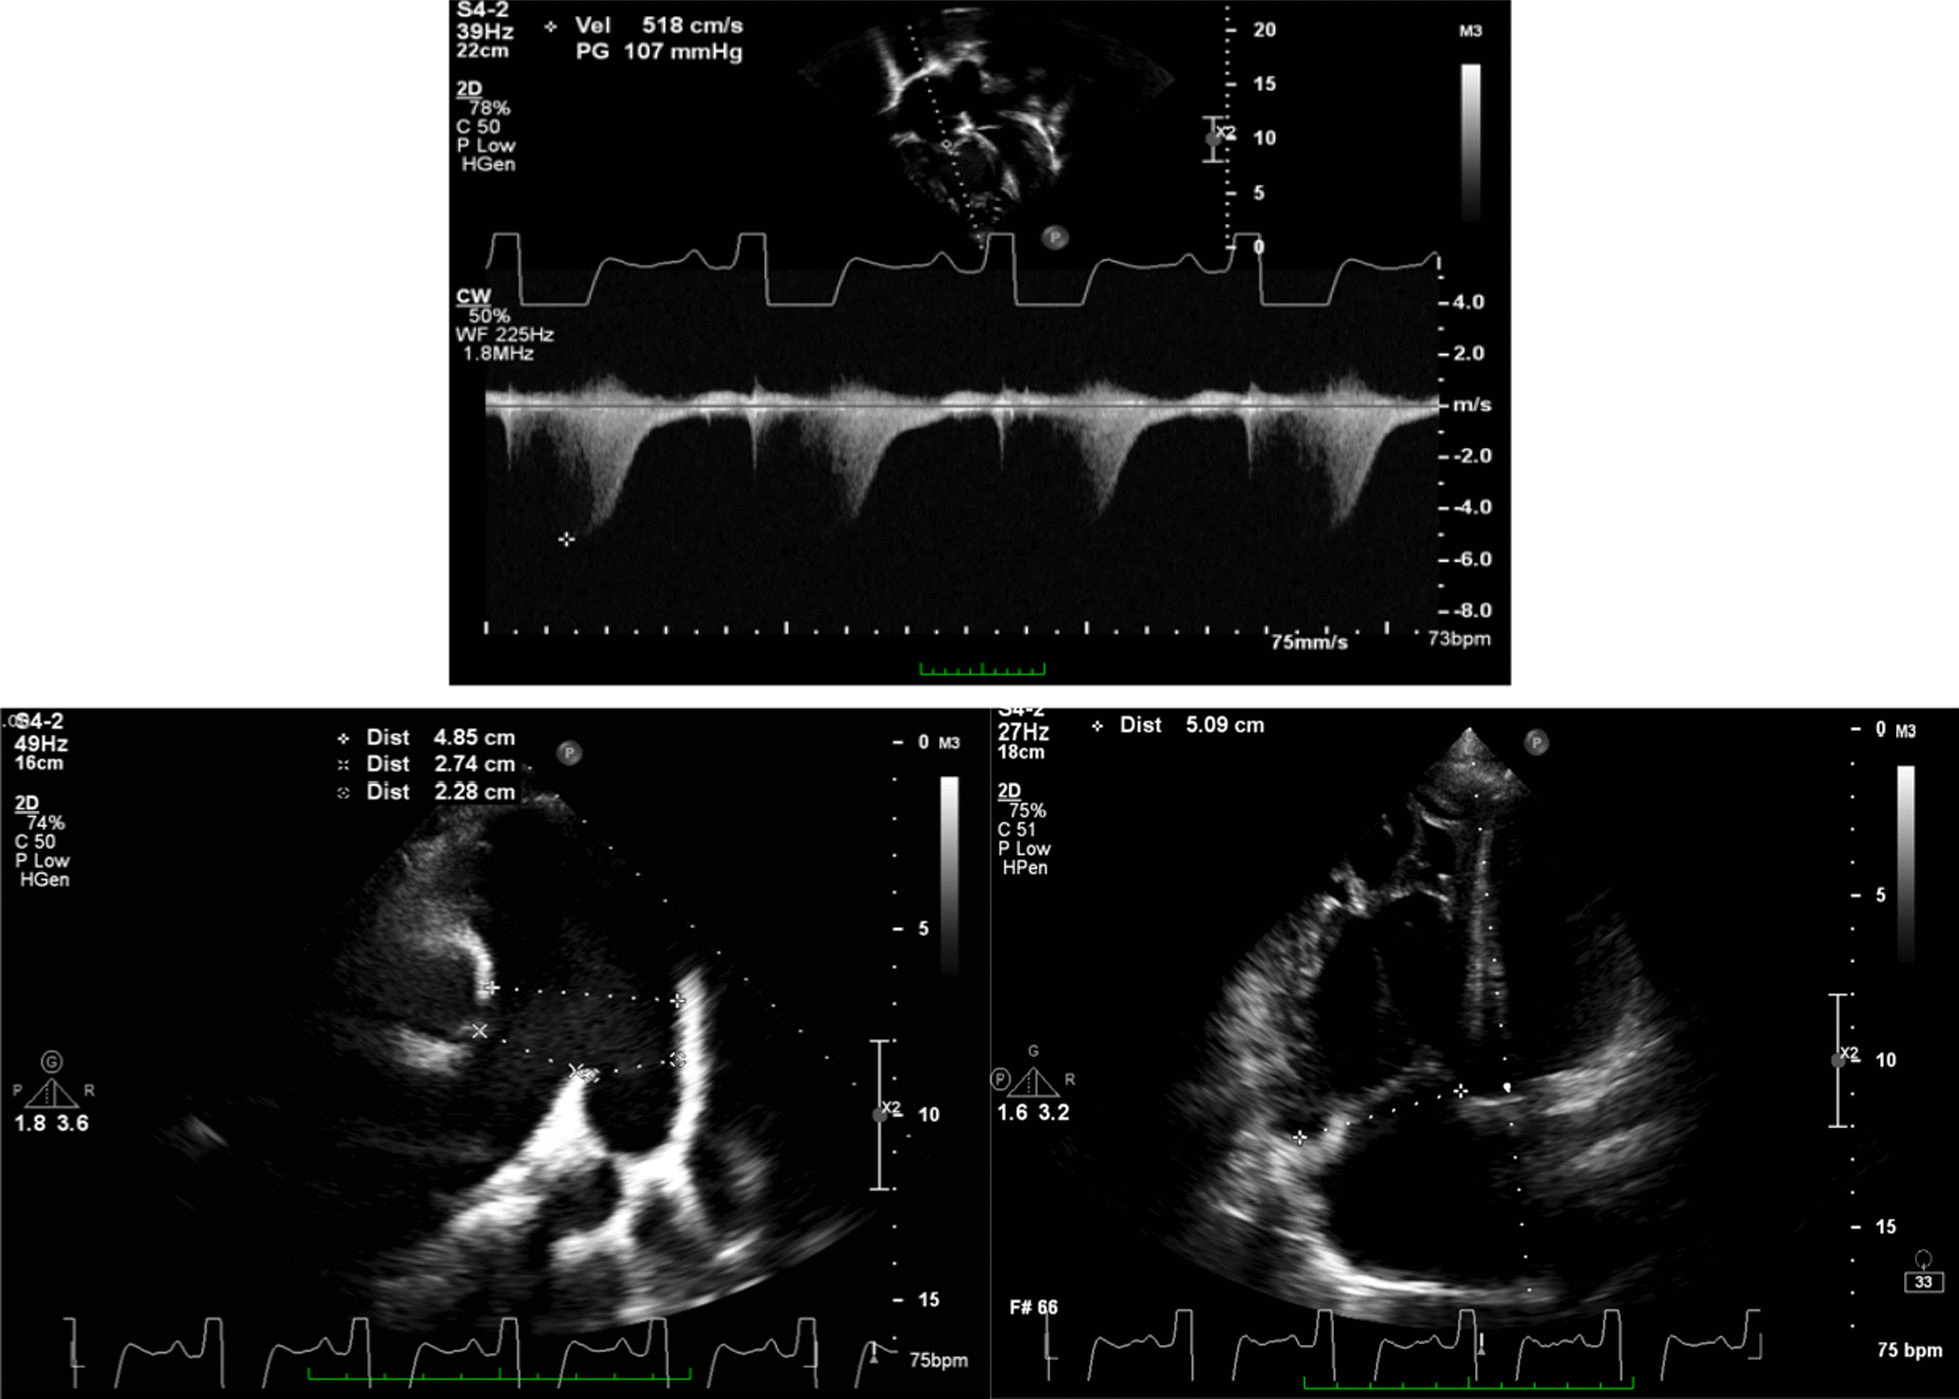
Transthoracic echocardiography images showed high probability of PH, (upper) with peak TR velocity of 5.1 m/s, (lower left) dilated MPA of 48 mm, (lower right) dilated RV of 51 mm
